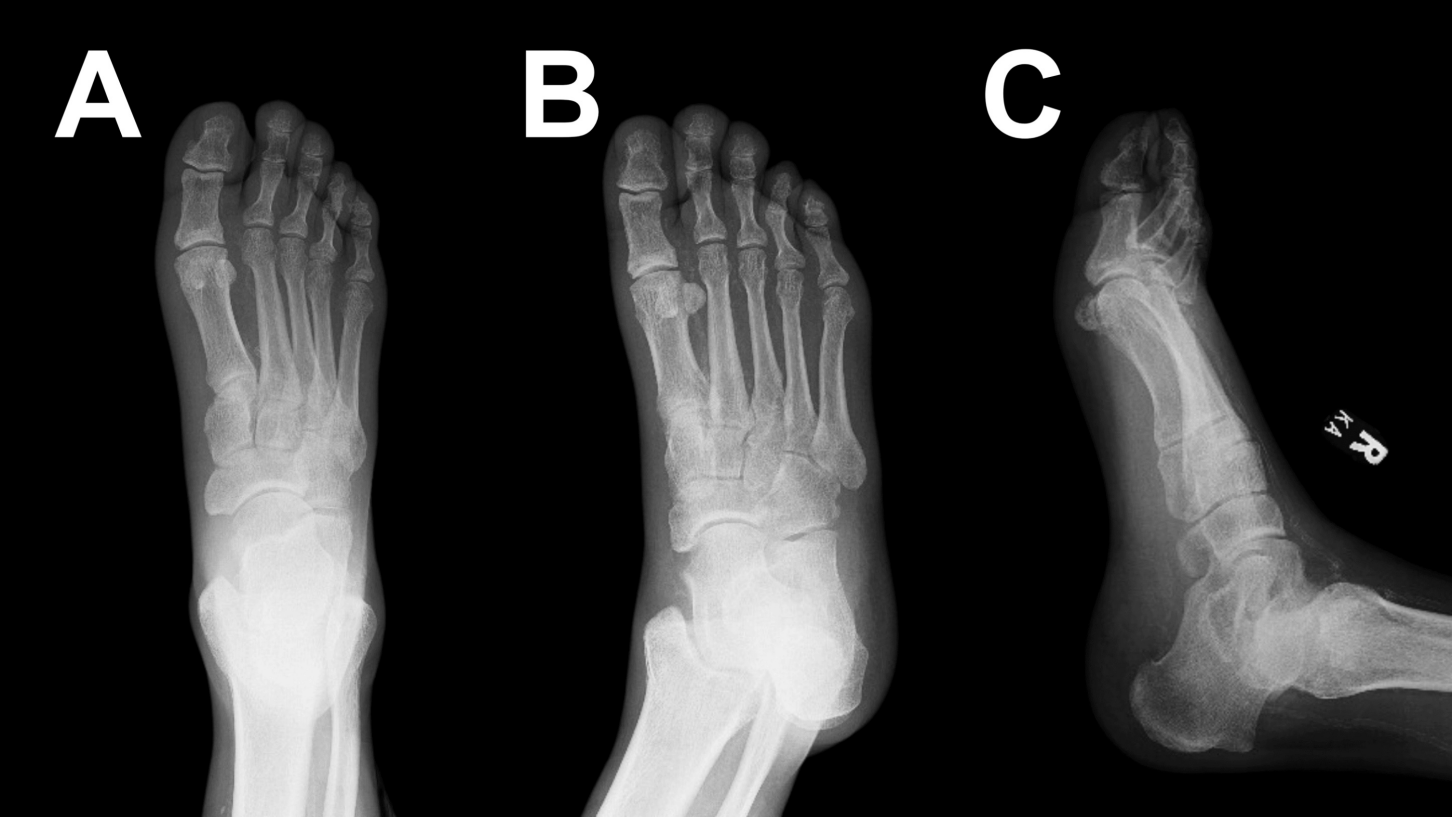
Anteroposterior (A), oblique (B), and lateral (C) plain radiographic imaging of the foot negative for acute fracture or subcutaneous air.
